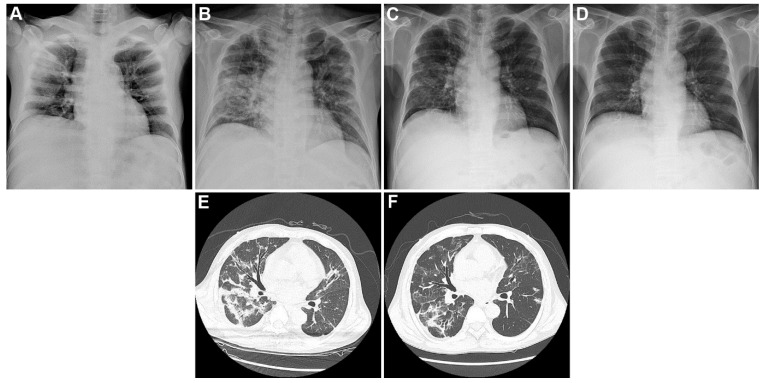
Temporal changes in chest imaging studies in one patient with end-stage renal disease and COVID-19. Temporal changes in chest radiography and computed tomography for patient number 2. Initial chest radiography upon admission showed focal consolidations in the right upper and left middle lung zones (A). (B,E) Images obtained on the 16th day of hospitalization showed bilateral worsening of multifocal patchy consolidations and ground glass opacities. (C,F) Images obtained on the 23rd day of hospitalization (the day of discharge) showed improvement of multifocal patchy consolidations and ground glass opacities, as well as organized changes. (D) Image obtained on 14th day after discharge (37 days from admission) showed resolved inflammatory lung lesions.
